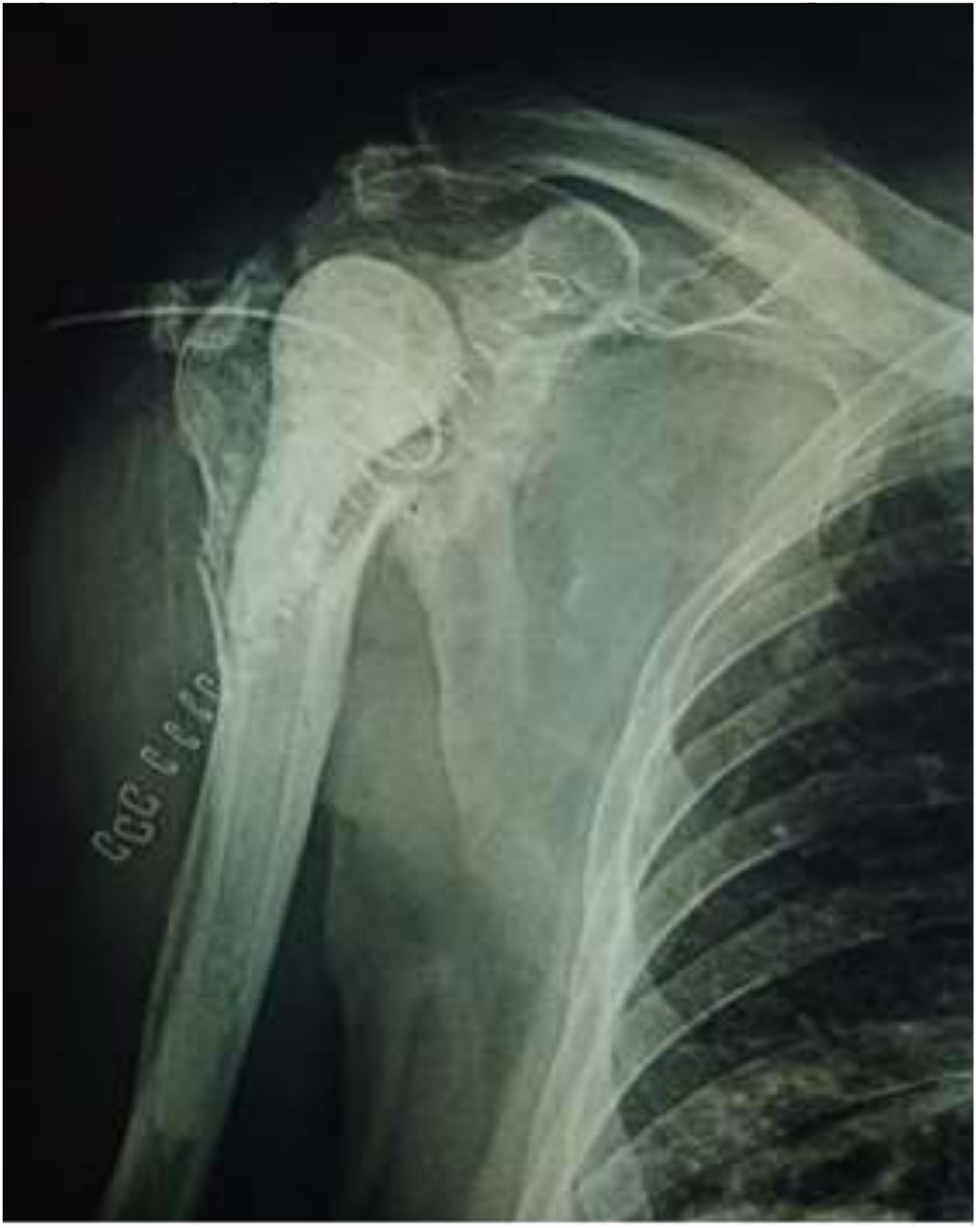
Radiograph of the shoulder in the final follow-up (30 months).
